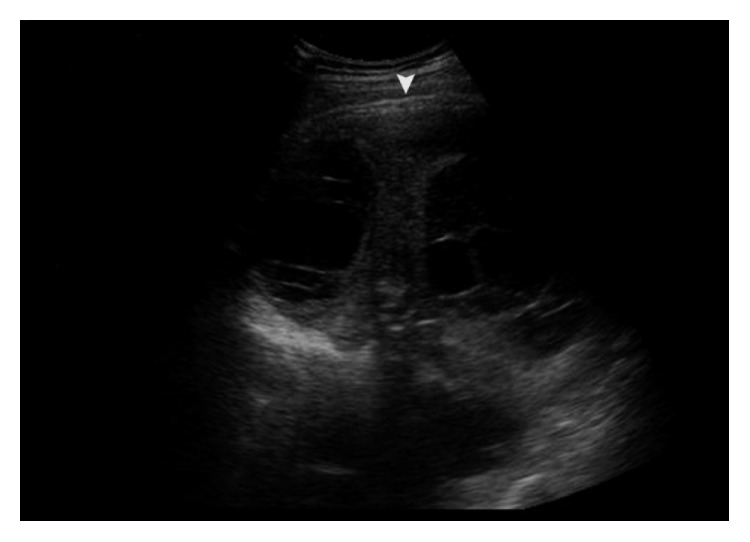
Chest sonography shows smooth pleural thickening (the arrow head) in complex septate effusion.
